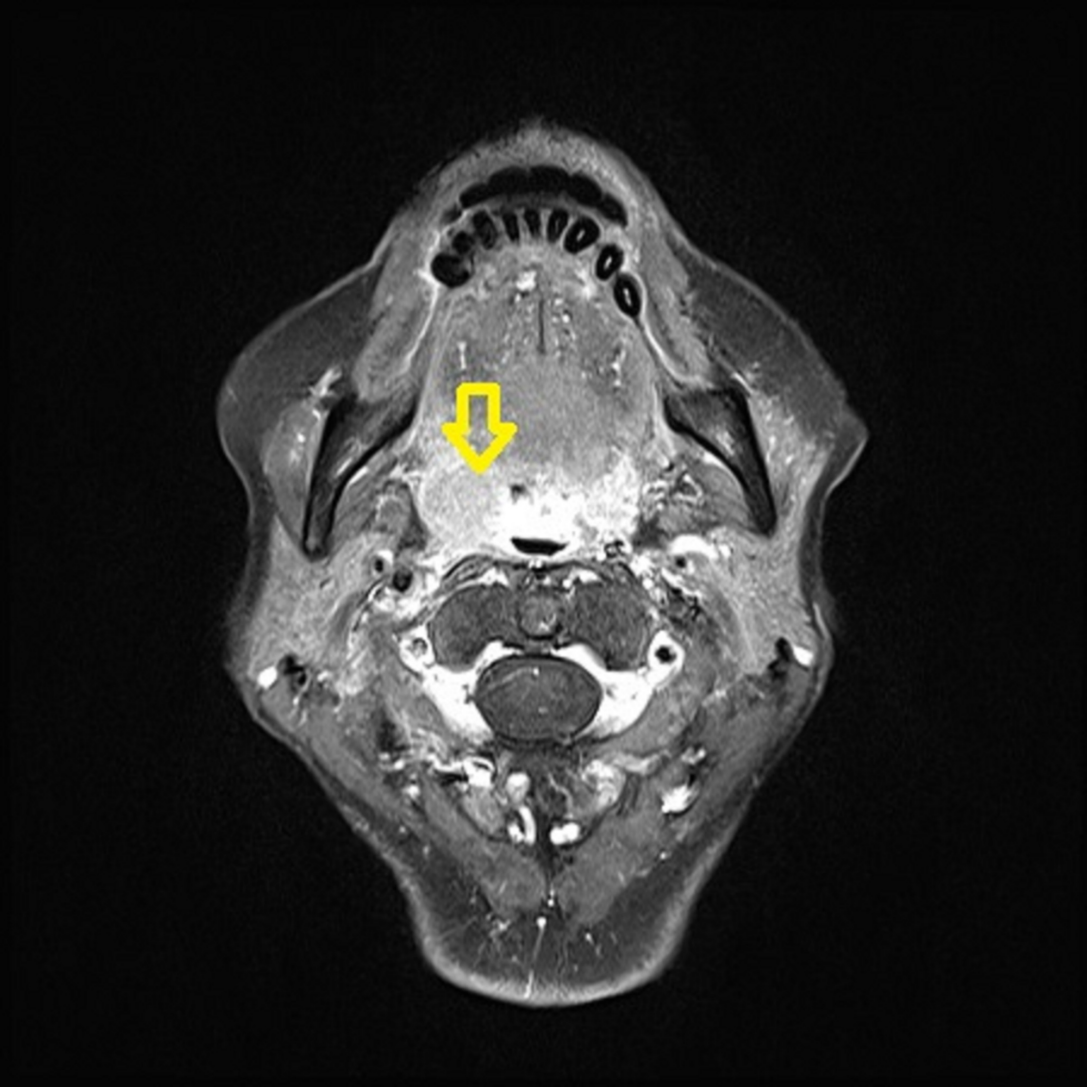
Case 1; head and neck MRI before CRT The tumor is located to the right in the oropharynx; size is about 3 cm MRI: magnetic resonance imaging; CRT: chemoradiotherapy
